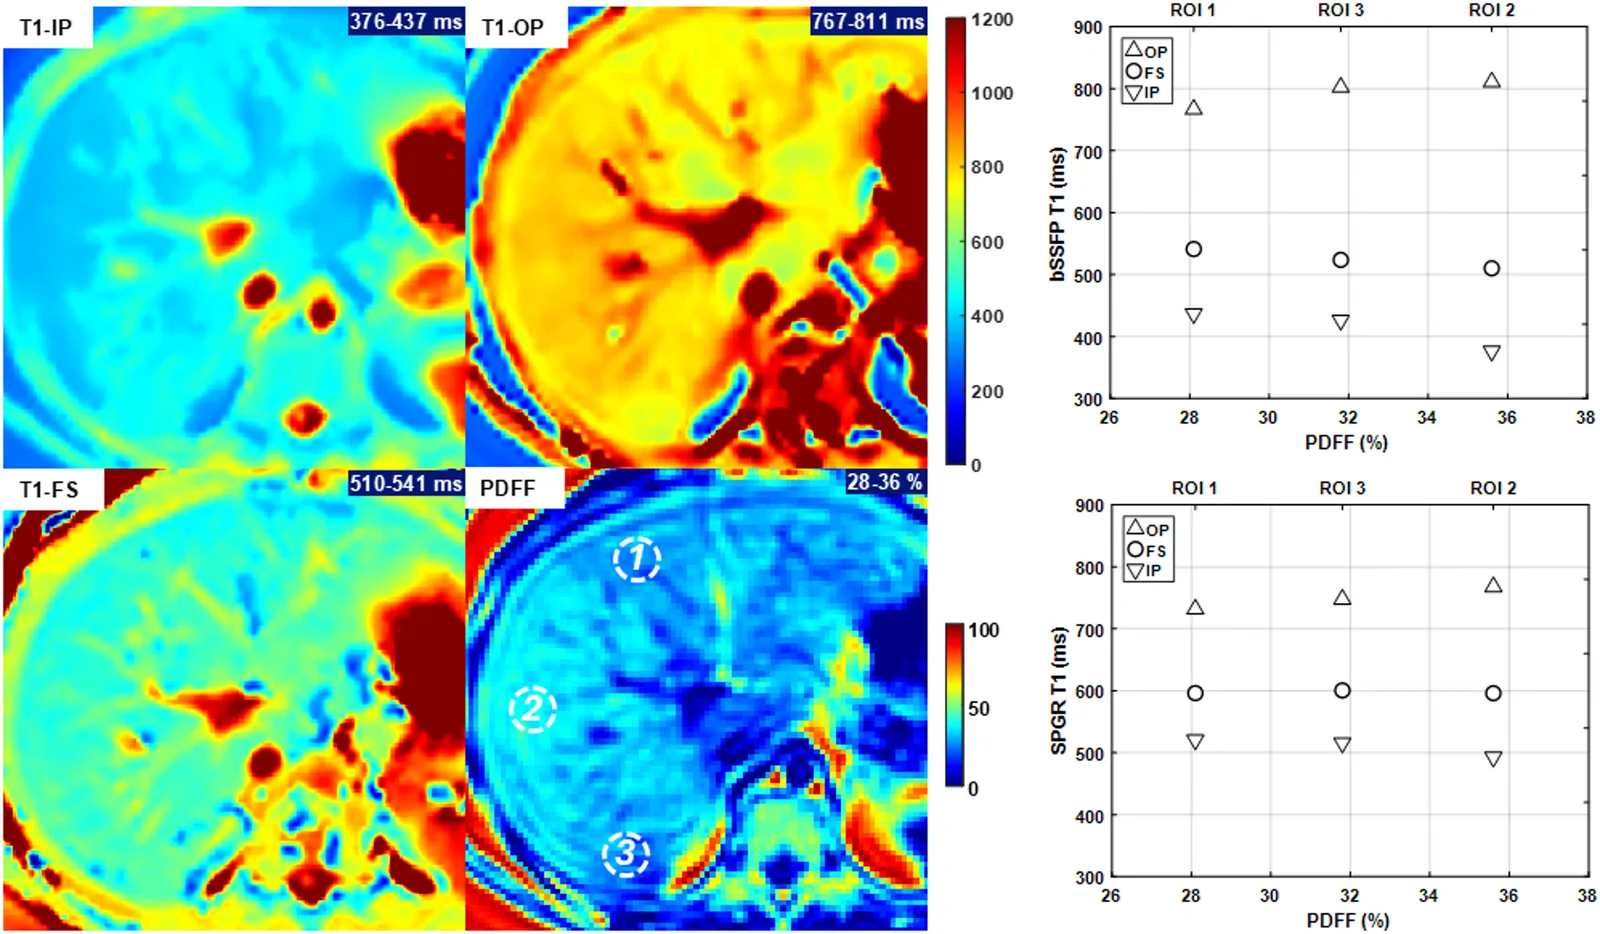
An 11-year-old male with elevated liver enzymes, ultrasound shear wave speed of 1.9 m/s, and clinical diagnosis of MASH demonstrated heterogeneous hepatic fat distribution, with PDFF ranging from 28% to 36% across three liver ROIs overlaid on the PDFF map. T1-IP, T1-OP, and T1-FS maps are shown from bSSFP MOLLI acquisitions. Corresponding bSSFP and SPGR T1 values acquired using IP, OP, and FS MOLLI acquisitions are shown in the adjacent scatter plots. T1-OP values increased with PDFF, while T1-IP decreased, and T1-FS remained relatively stable across ROIs. The marked difference in T1-OP between the right lateral (ROI 2) and right anterior (ROI 1) liver segments reflects the TE-dependent influence of fat on MOLLI-based T1 estimation
